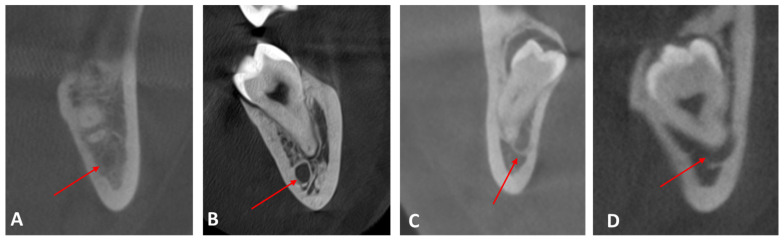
CBCT images demonstrating different contact relationships between the mandibular third molar and the mandibular canal: (A) No contact; (B) Contact with a complete white line; (C) Contact with a defective white line; (D) Penetration of the mandibular canal. Red arrows indicate the mandibular canal.
